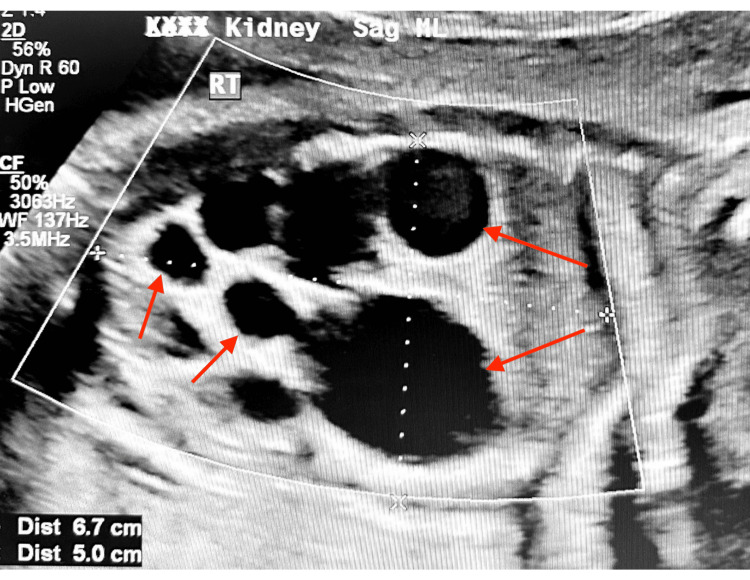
Fetal ultrasound scan of the kidney at 24 weeks, one day of gestation Arrows show the enlarged right multicystic kidney with the largest cyst measuring 5.3 x 3.2 x 2.9 cm.
